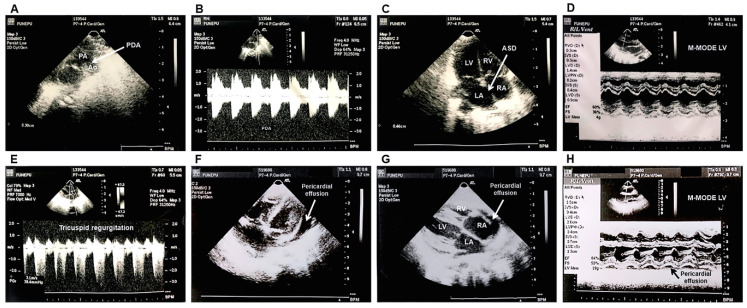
Echocardiographic assessment showing the presence of the patent ductus arteriosus (PDA) and interatrial communication 14 days after birth (A–E) and pericardial effusion in the last hospitalization of the 19 month old girl (F–H): (A,B) Transversal view indicating the PDA of 3.8 mm and Doppler showing a systodiastolic flow through the PDA, respectively. (C,E) Apical view indicating atrial septal defect (ASD) and tricuspid regurgitation with pulmonary hypertension respectively. (D) M-mode echocardiogram showing preserved left ventricular function. (F,G) Apical and subcostal view respectively indicating the pericardial effusion. (H) Transversal view showing a pericardial effusion of 7.0 mm and preserved left ventricular function. Legend: Ao: aorta. ASD: atrial septal defect. LA: left atrium. LV: left ventricle. PA: pulmonary artery. PDA: patent ductus arteriosus. RA: right atrium. RV: right ventricle.
